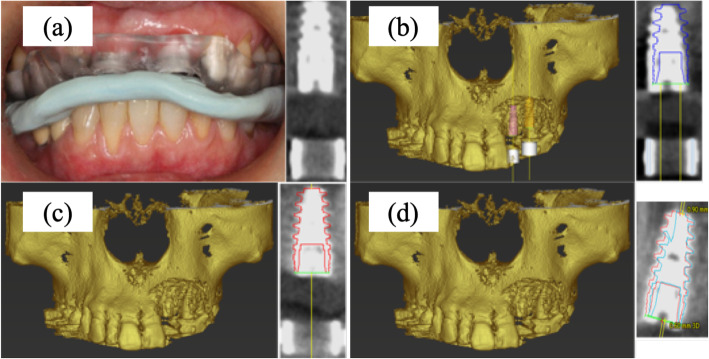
Evaluation method of implant validation. a Immediately after the implant installation, the surgical guide used for the surgery was attached, and a cone-beam computed tomography scan was performed with the bite index prepared in advance to stabilize the position. b The position of the planned implant body was specified from the position of the metal sleeve in the jaw bone model, and implant model 1 was installed there. c Implant model 2 was placed according to the implant body actually inserted. d The deviation between the implant models was measured three-dimensionally on the jaw bone model
